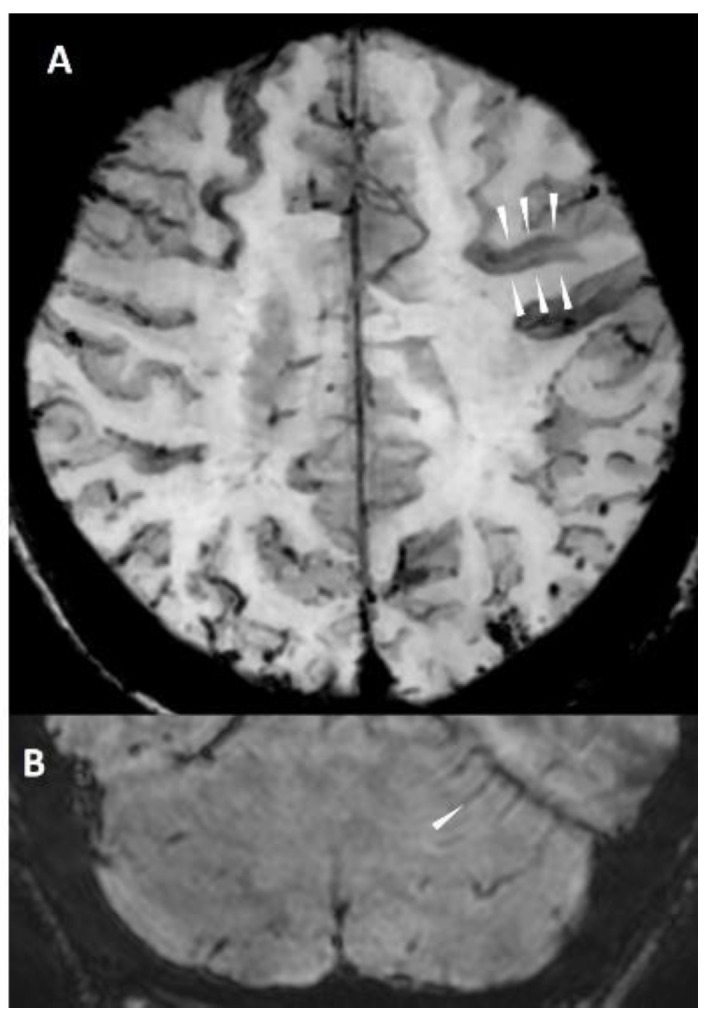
Cortical superficial siderosis. The coating of both surfaces of the sulcus can be appreciated ((A), white arrowheads) on axial venBOLD MRI (3T, TE = 25 ms, TR = 18 ms, 10 mm MinIP). Infratentorial siderosis along the folia of the cerebellum ((B), white arrowhead) on axial venBOLD MRI (1.5 T, TE = 49 ms, TR = 34 ms, slice thickness 2.2 mm).
